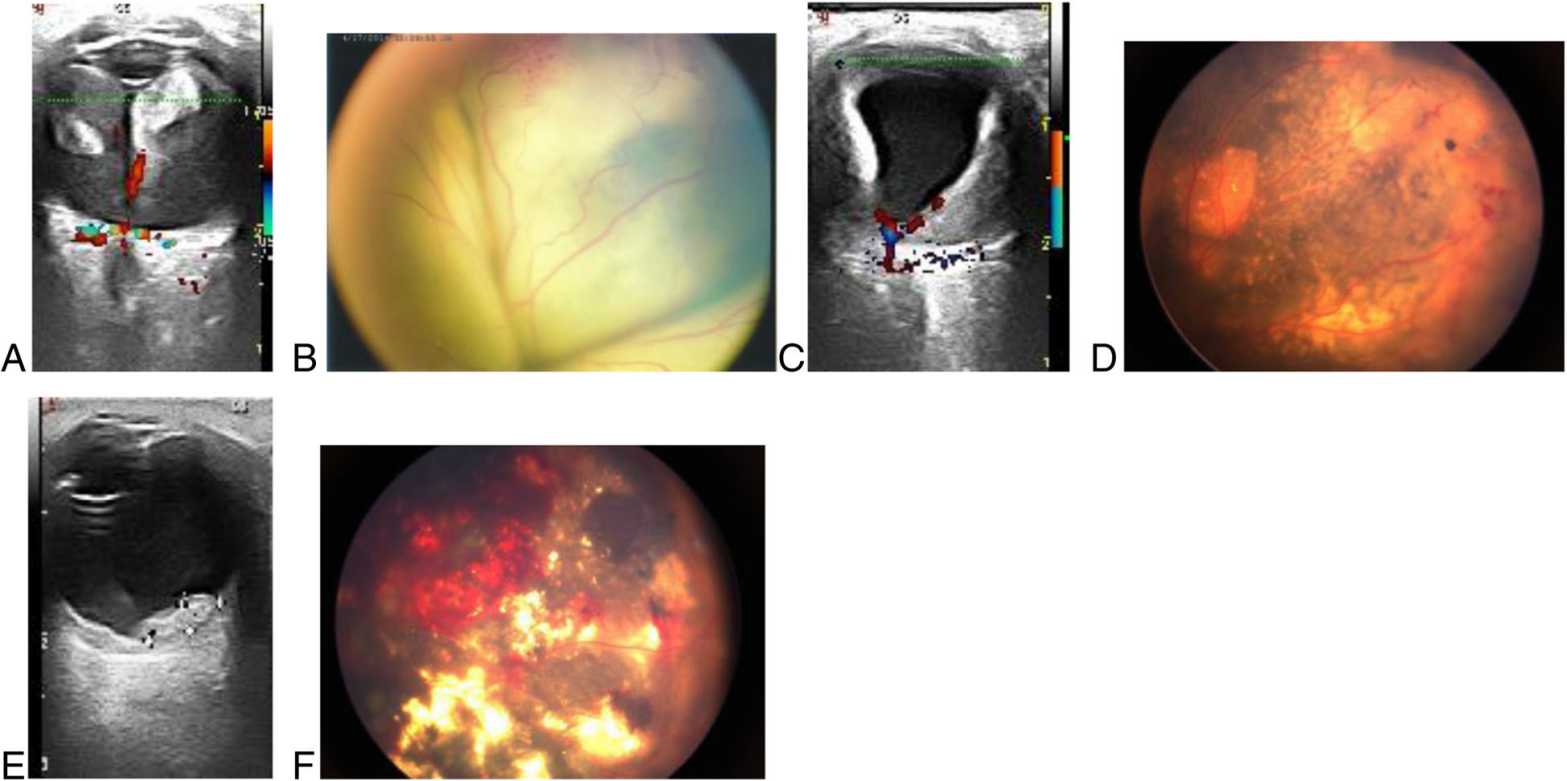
3-year-old boy with Coats’ disease underwent 3 IVR and 1 laser sessions.The preoperative Retcam images and Color Doppler ultrasound images showed total retinal detachment at baseline (a, b). After 1 month and 3 months of treatment,the Retcam images and Color Doppler ultrasound images showed absorption of Subretinal effusion gradually (c, d), and reattachment of retinal (e, f)
